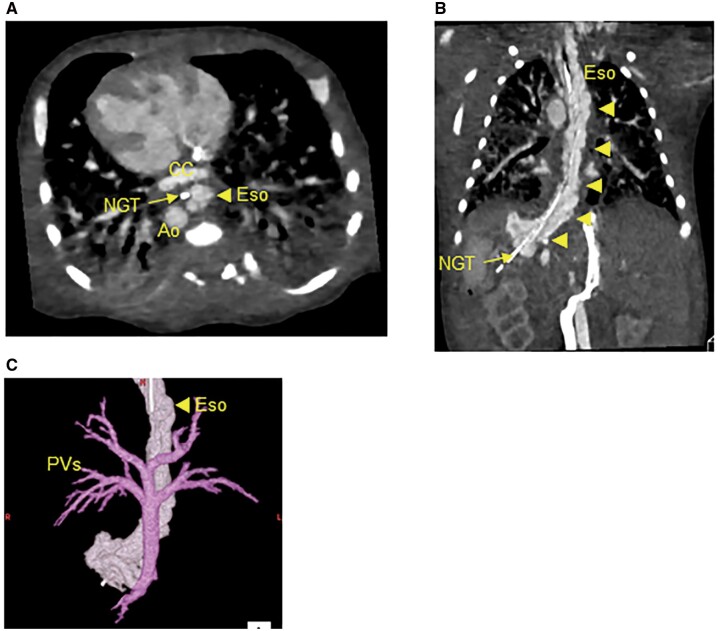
Remarkably dilated oesophageal varices in preoperative computed tomography. (A) The axial image, (B) the coronal image and (C) the constructed 3D image of preoperative computed tomography. Arrowheads indicate the oesophageal varices. Ao: aorta; CC: common chamber; Eso: oesophagus; NGT: nasogastric tube; PV: pulmonary vein.
